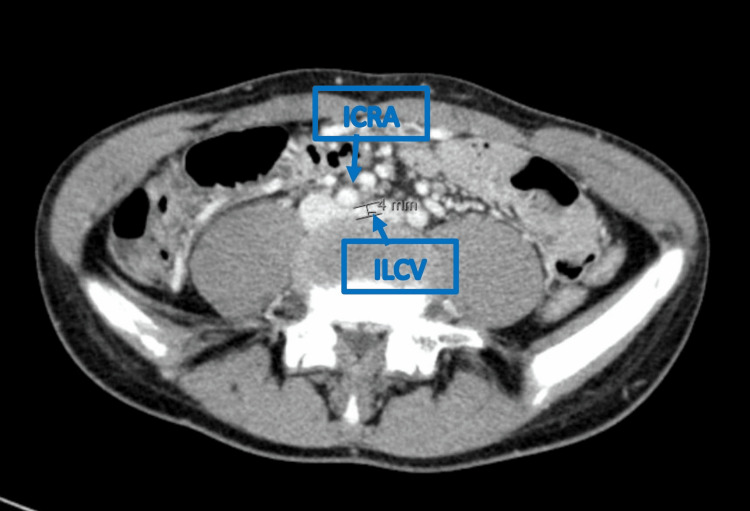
Axial Computed Tomography (CT) scan Axial abdominal CT scan demonstrating compression of the left common iliac vein (ILCV) by the overlying right common iliac artery (ICRA) against the vertebral body, resulting in venous narrowing. Findings are consistent with May-Thurner syndrome.
